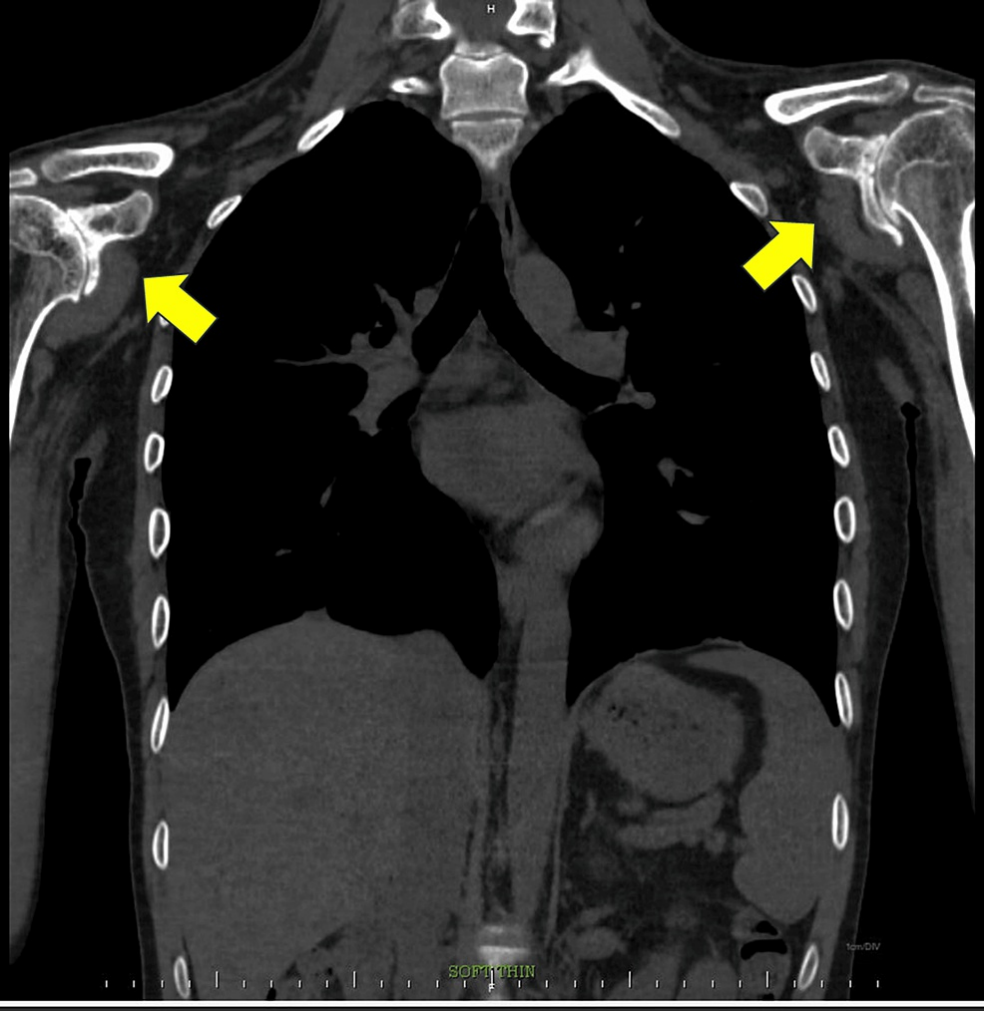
HRCT thorax: coronal oblique view No pleuro-parenchymal abnormalities were noted. Both the yellow arrows depict bilateral glenohumeral joints showing degenerative changes in the form of loss of humeral head contour, joint narrowing, marginal osteophytes, subchondral sclerosis, and marked osteopenia. Early changes of pseudoarthrosis were noted. HRCT thorax: high-resolution computed tomography of the thorax
